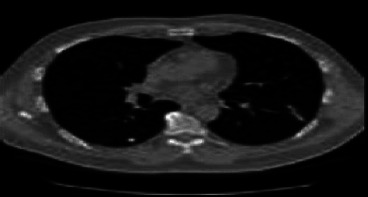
CT image that has been preprocessed.
